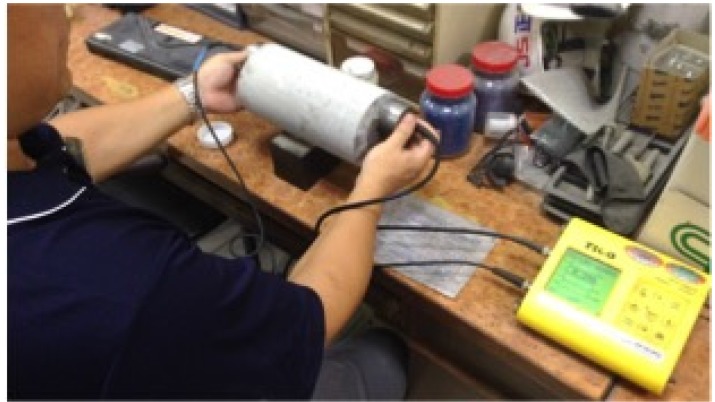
Ultrasonic pulse velocity test.
